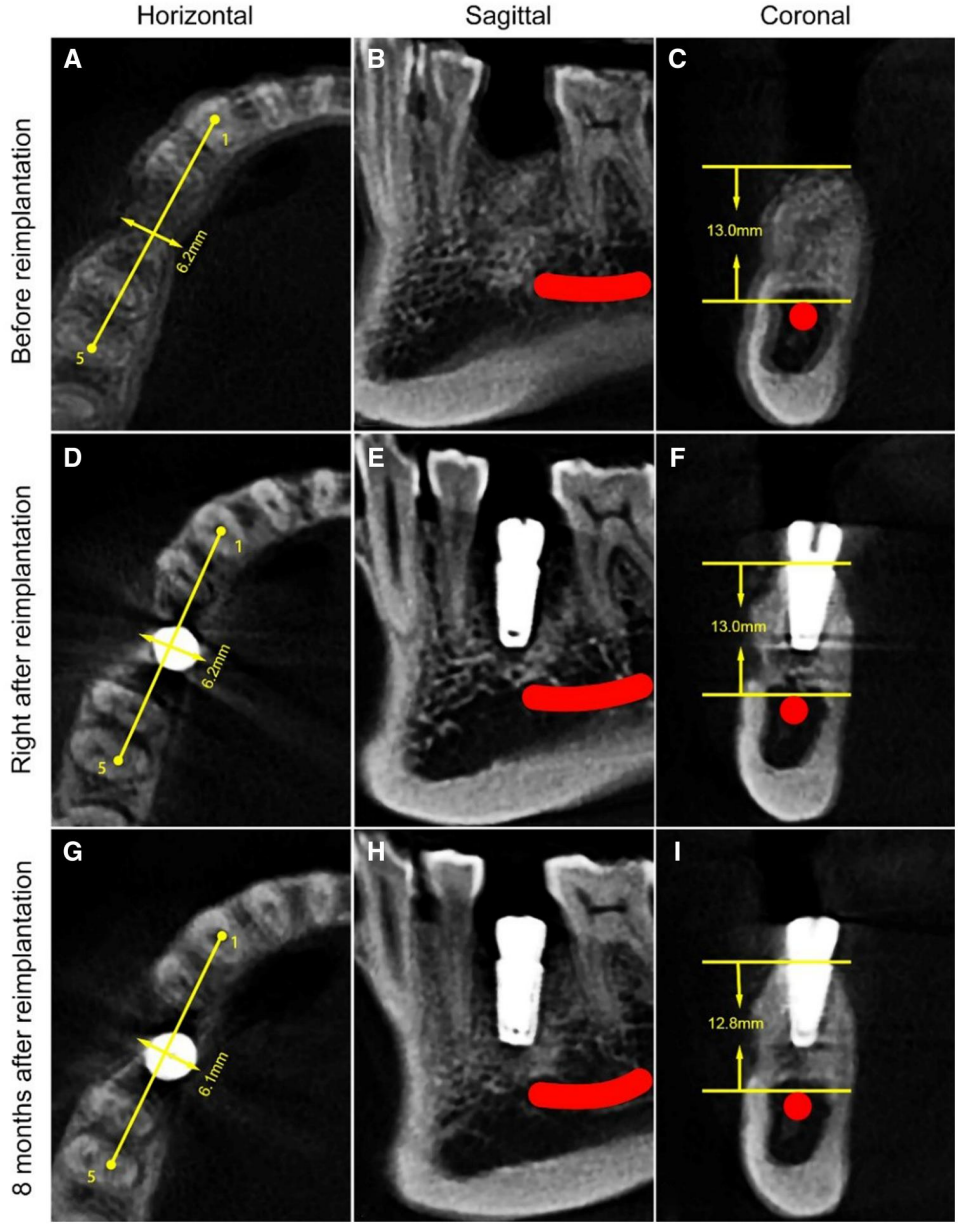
CBCT Images. (A–C) Before reimplantation (15 months after bone grafting), (D–F) immediately after reimplantation; (G–I) 8 months after reimplantation. Red line and dot indicate the mandibular nerve canal.
